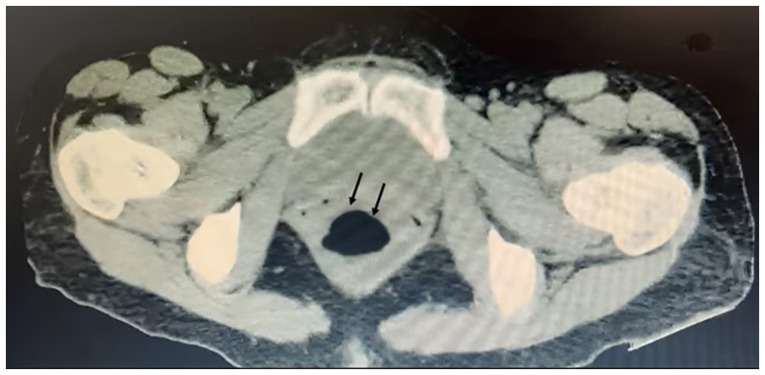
Abdominal computed tomography scan. An hypogenic mass located in the region posterior to the uterus of the patient is shown (black arrows).
